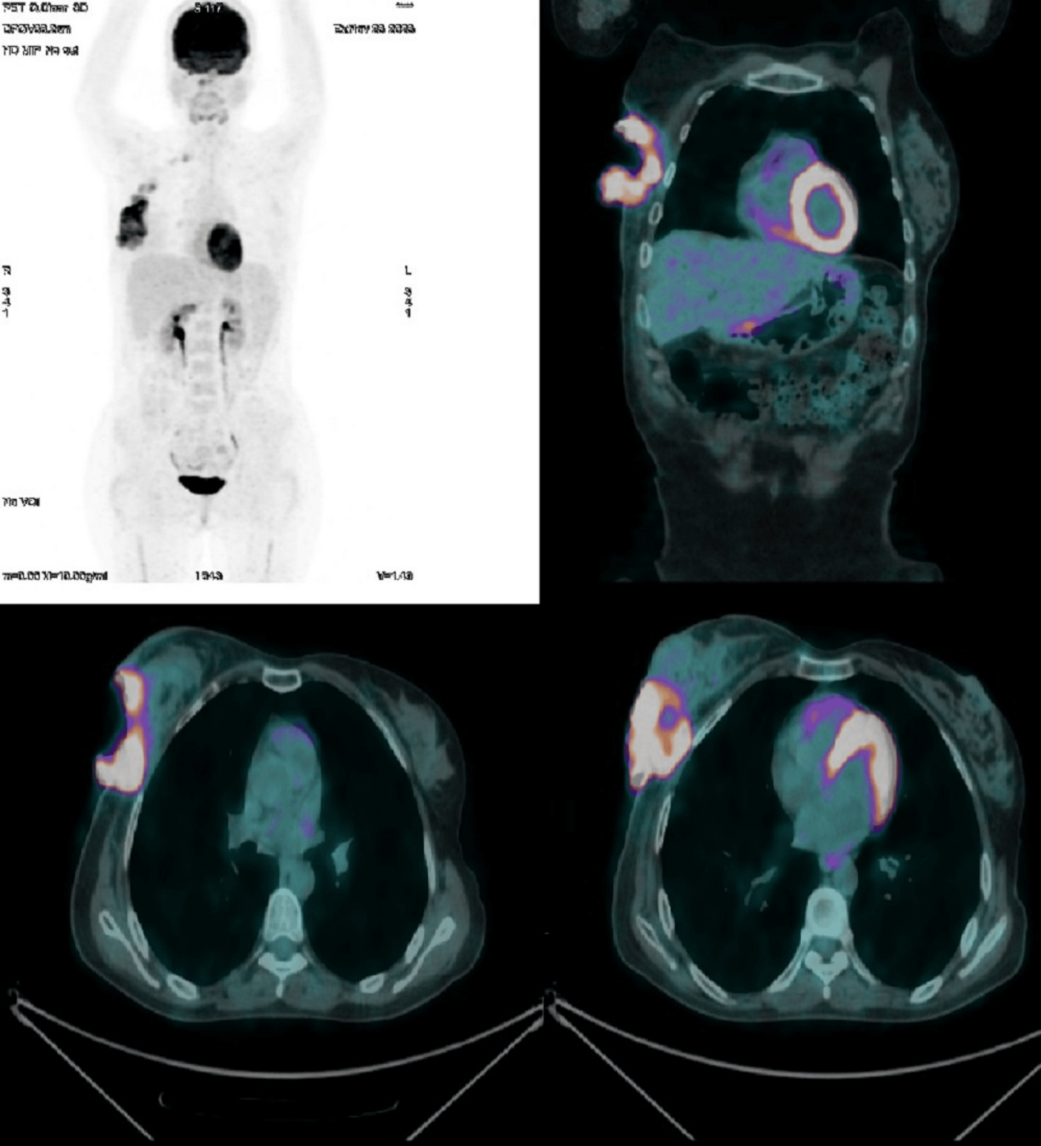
Axial and coronal reconstructions of PET scan (18F-FDG) demonstrating hypermetabolism of a large right breast mass (SUV 30) without evidence of distant adenopathy or secondary lesions. FDG: fludeoxyglucose; SUV: standardized uptake value
